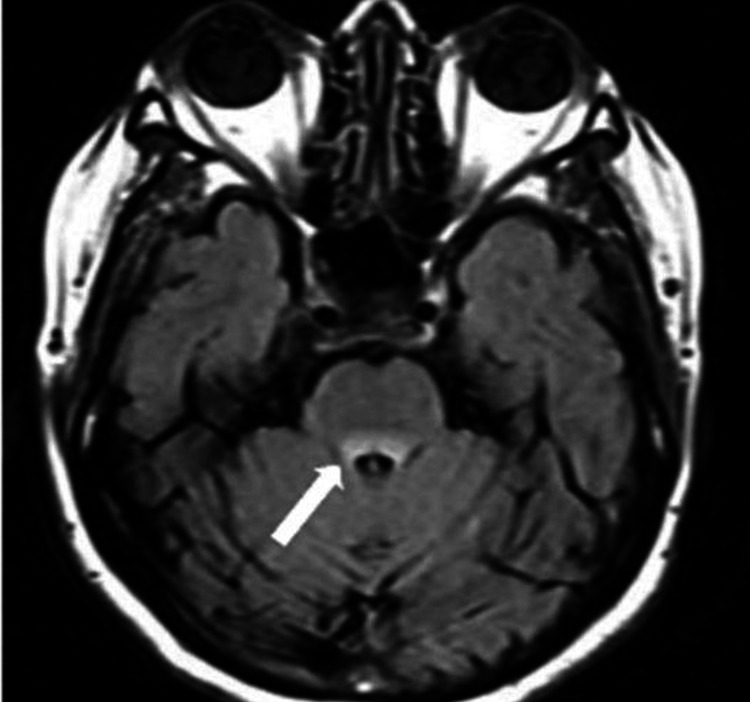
Axial view of brain MRI showing the mesencephalic tegmentum signal abnormality on FLAIR MRI: magnetic resonance imaging; FLAIR: fluid-attenuated inversion recovery
